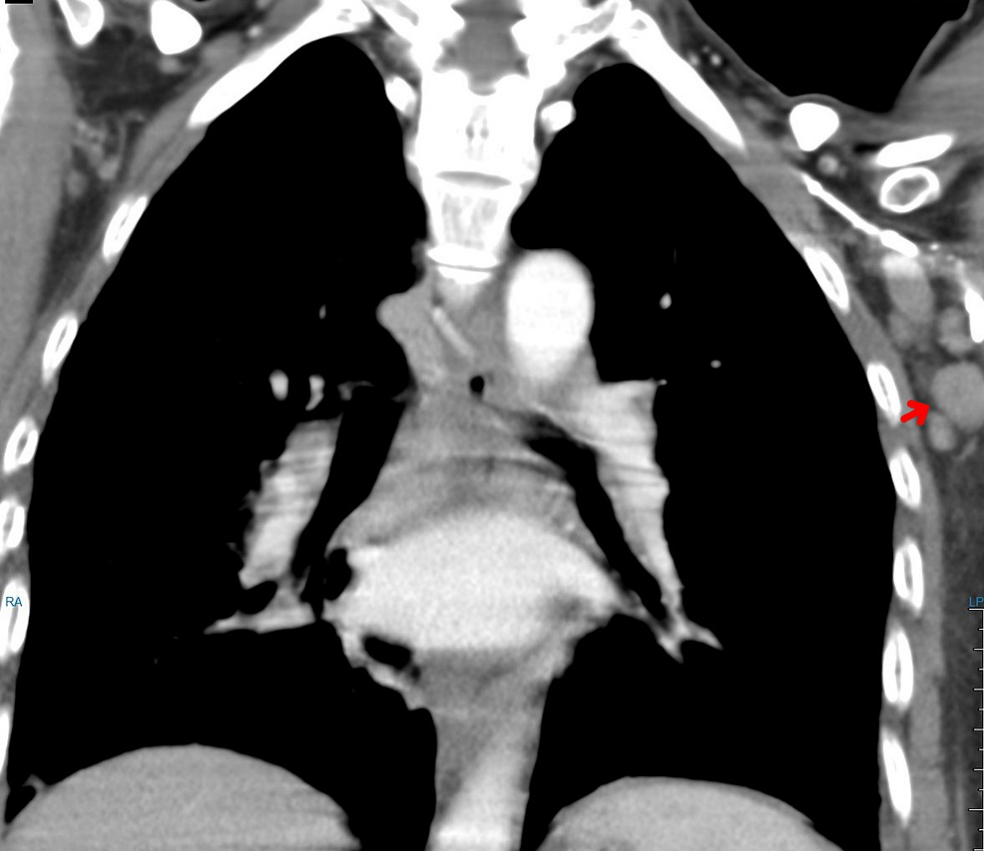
Chest CT (February 17, 2016) showing enlarged left axillary lymph node of 2.5 cm
